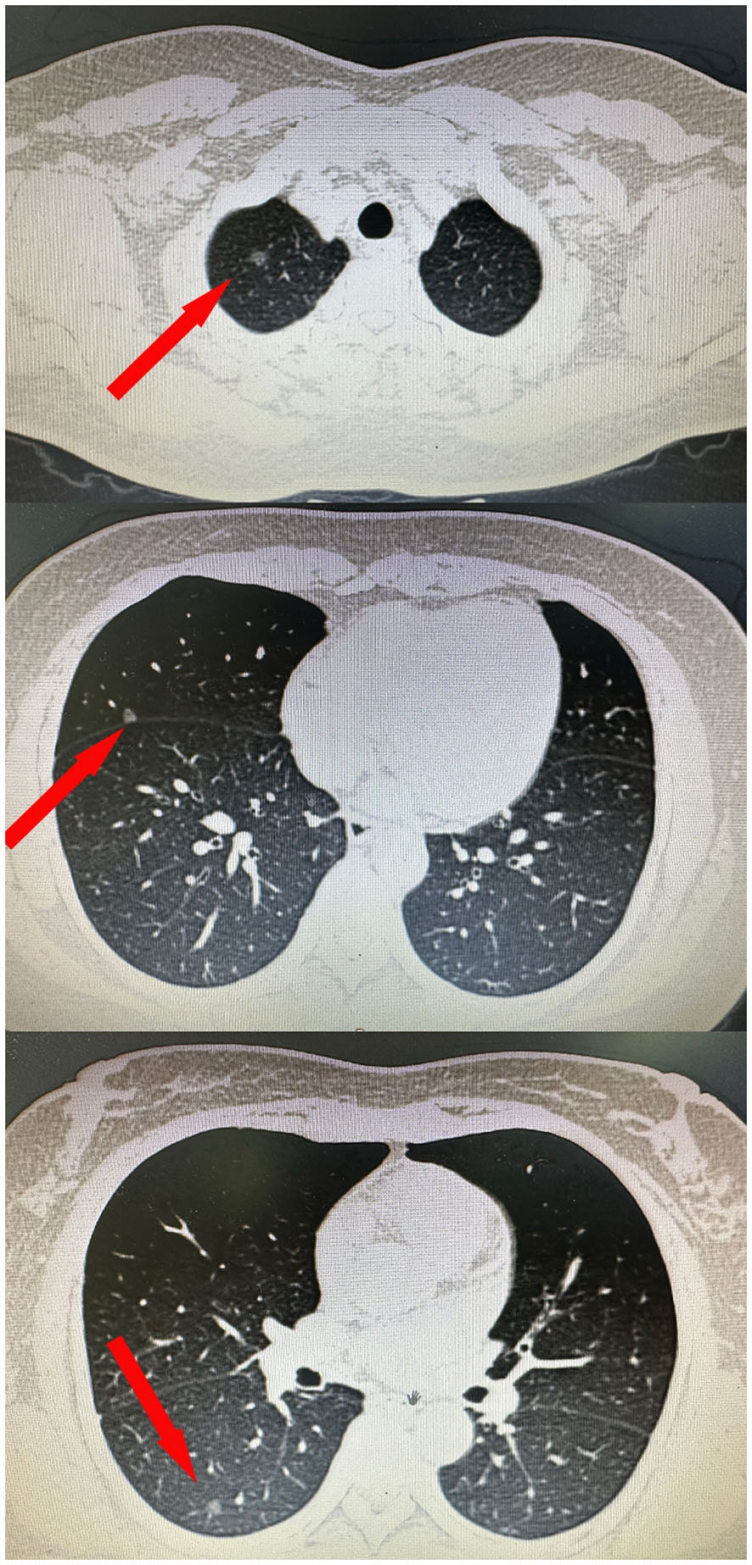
CT data of the patient’s three pulmonary nodules.
